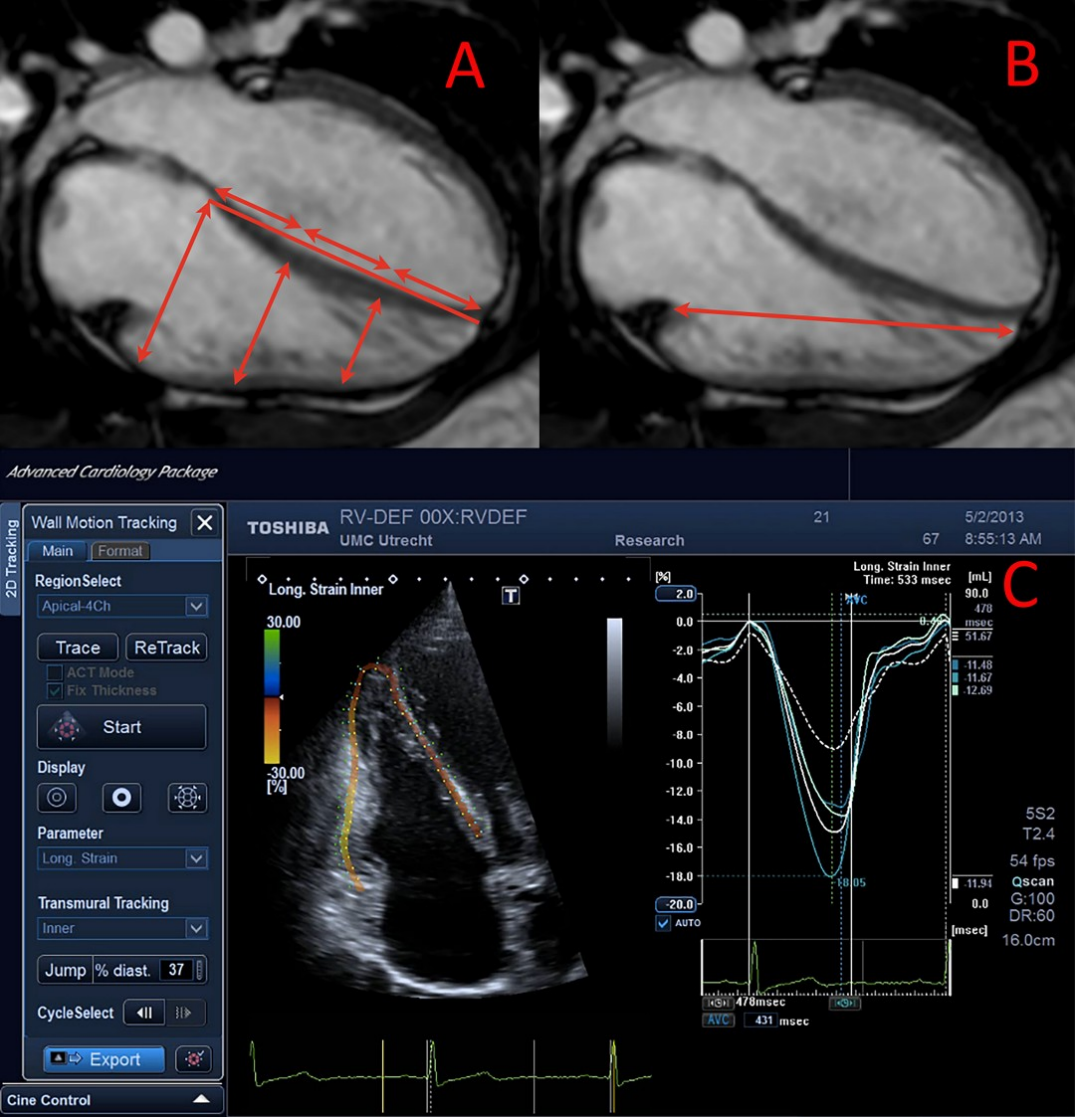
An example of cardiac magnetic resonance and echocardiographic analyses. A&B: Image of CMR analysis for transverse motion (a) perpendicular to the apex-base axis and of longitudinal motion (b). C: Image of longitudinal deformation analysis of the RV.
